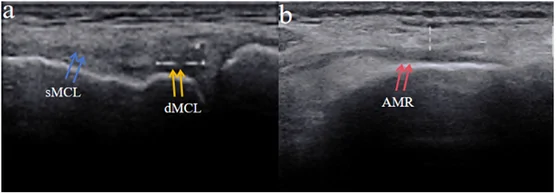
(a) Ultrasound imaging of the medial knee structures shows the sMCL (blue), the dMCL (yellow). (b) Ultrasound imaging of the AMR (red). (AMR, anteromedial retinaculum; dMCL, deep medial collateral ligament; sMCL, superficial medial collateral ligament.)
